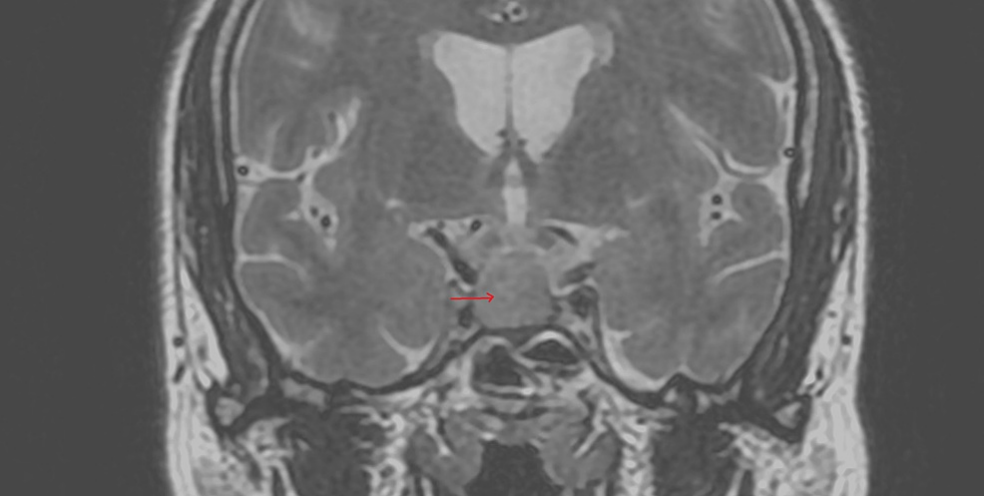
Pituitary MRI with gadolinium contrast; coronal T2W image showing a pituitary macroadenoma of 16x16x12 mm diameter
